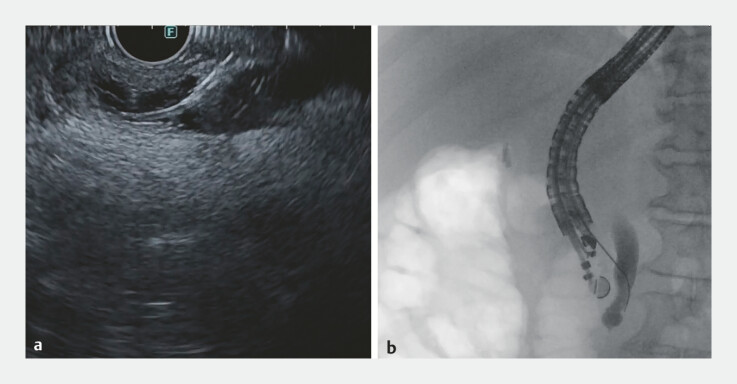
EUS-guided puncture of the extrahepatic bile duct (left); cholangiography confirming correct position of the 19-gauge needle (right). Abbreviation: EUS, endoscopic ultrasound.
